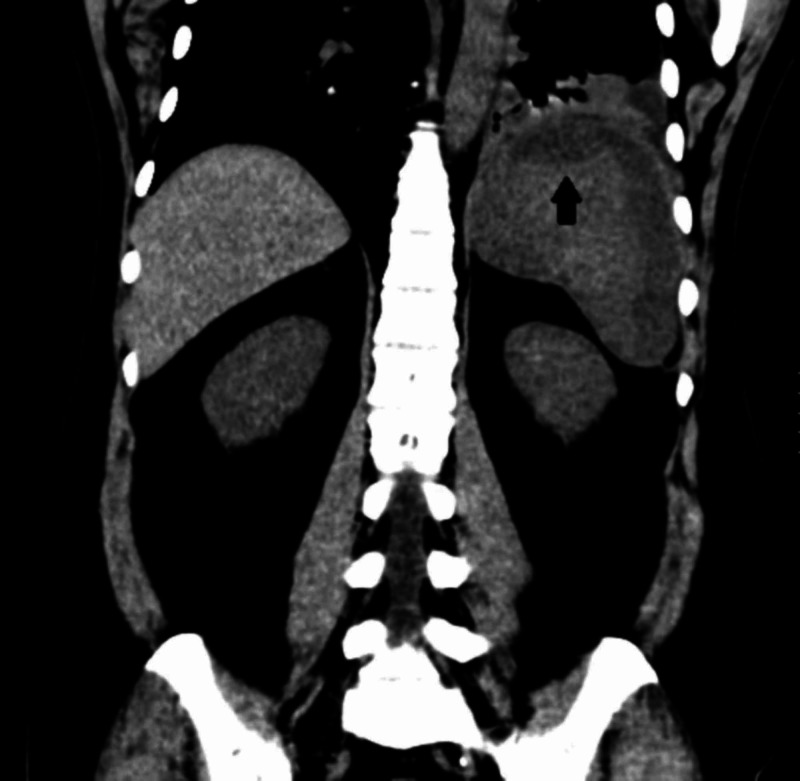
CT of the abdomen Coronal CT of the abdomen showing splenomegaly and low attenuated images in the spleen compatible with splenic infarction.
